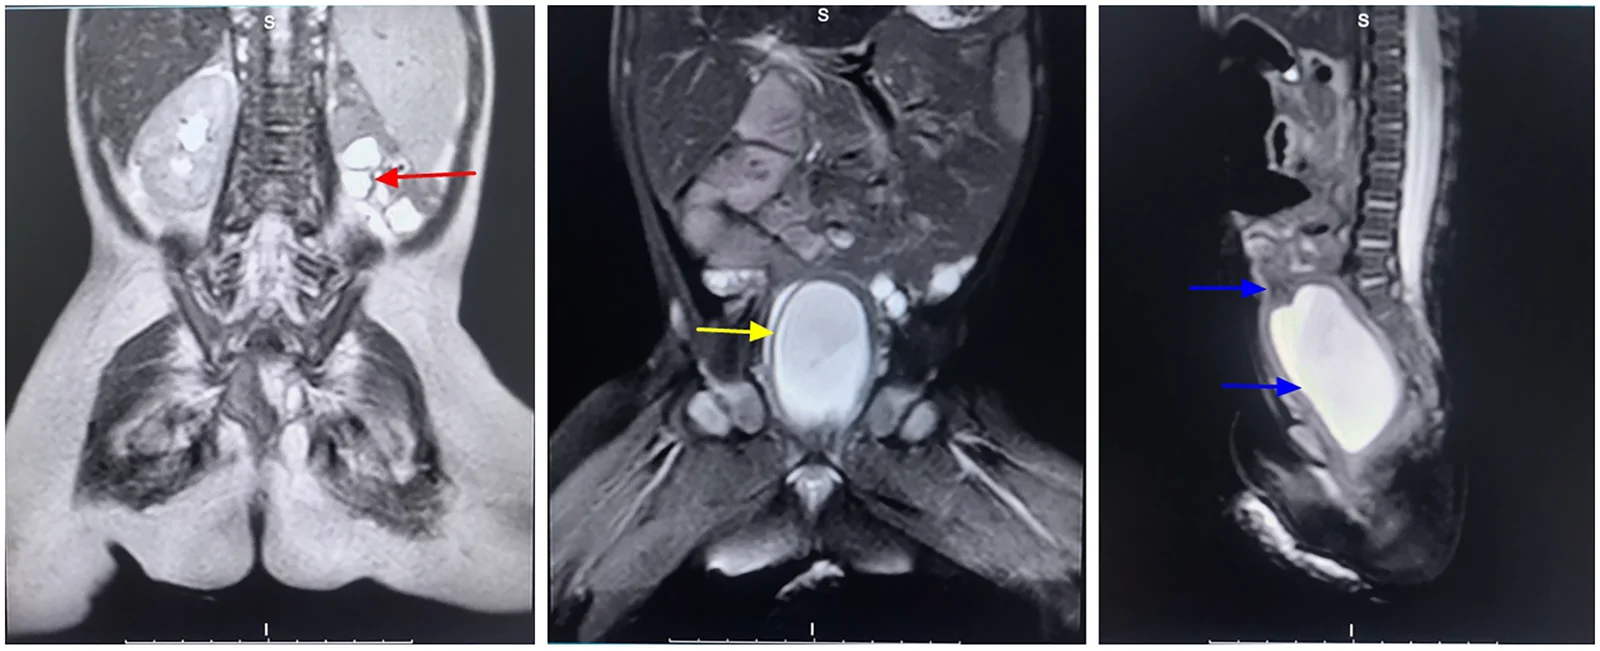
MRI findings: Left-sided polycystic kidney with dysplasia (red arrow) (A); formation of a left vaginal septum with associated vaginal effusion (yellow arrow) (B); bilateral cervical abnormalities and significant vaginal fluid accumulation (blue arrow) (C).
